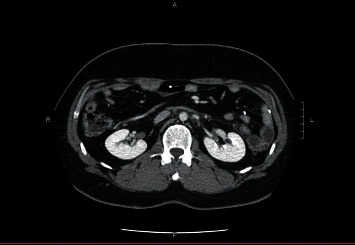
CT imaging of the abdomen and pelvis reveals significant improvement in retroperitoneal mass appearance at 12 weeks.
